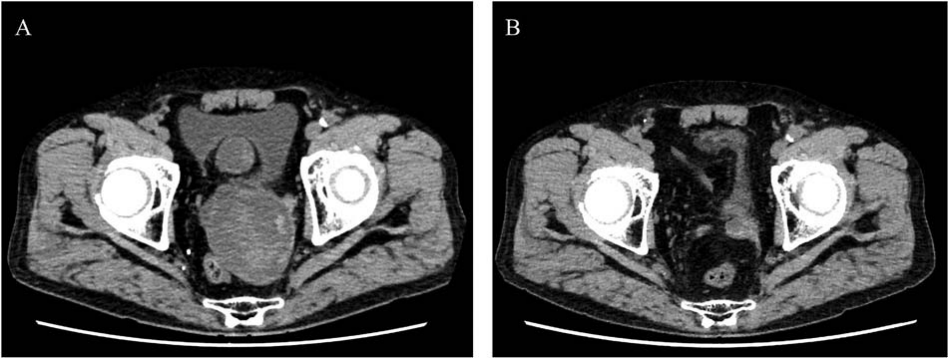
Postoperative computed tomography showing a reduction in aneurysm size. A—Preoperative image, B—10-month postoperative image.
